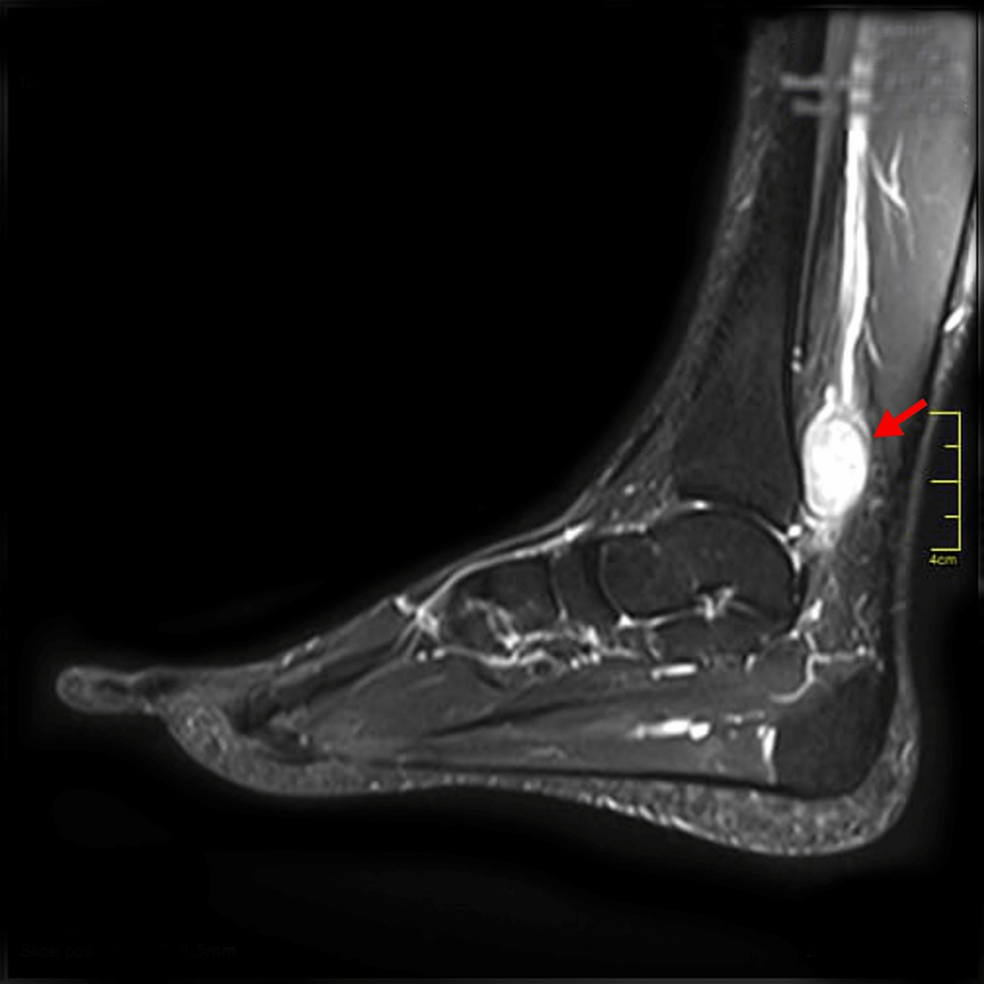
Magnetic resonance imaging (sagittal view) with contrast of the patient's left foot showing hyperintense ovoid mass (red arrow)
